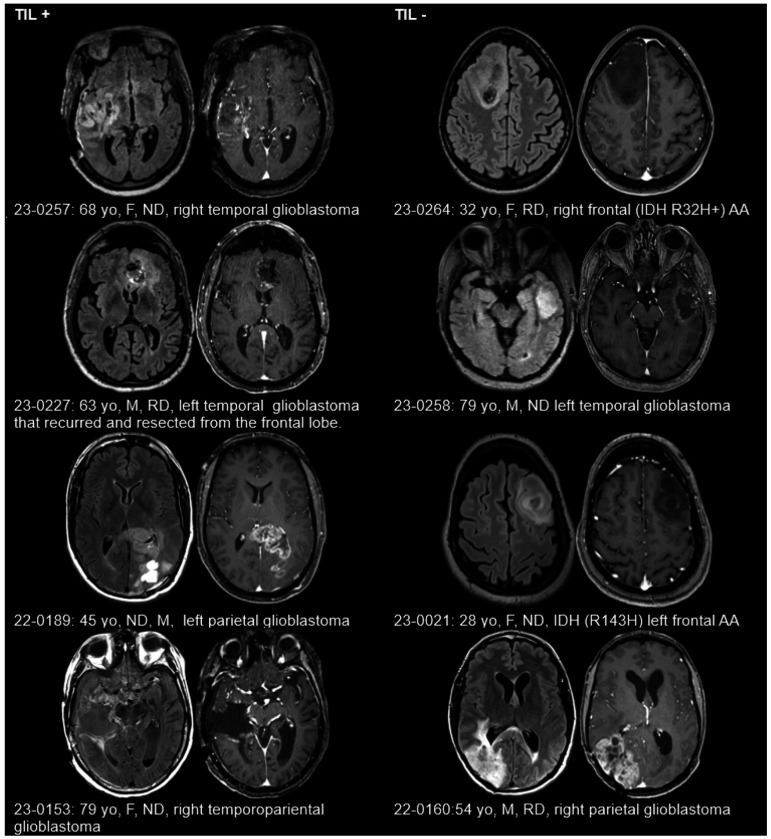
Clinical and MRI Characteristics of eight glioma patients arranged by Tumor-Infiltrating Lymphocyte (TIL) expansion success during *ex vivo* expansion with IL-2. For each patient, axial T2-weighted FLAIR (left) and T1-weighted post-contrast (right) images are presented, highlighting tumor location and imaging features. Abbreviations: F: Female, M: Male, R: Recurrent Disease, ND: Newly Diagnosed Disease. IDH: Isocitrate Dehydrogenase mutations: AA: Anaplastic Astrocytoma, yo: years old.
